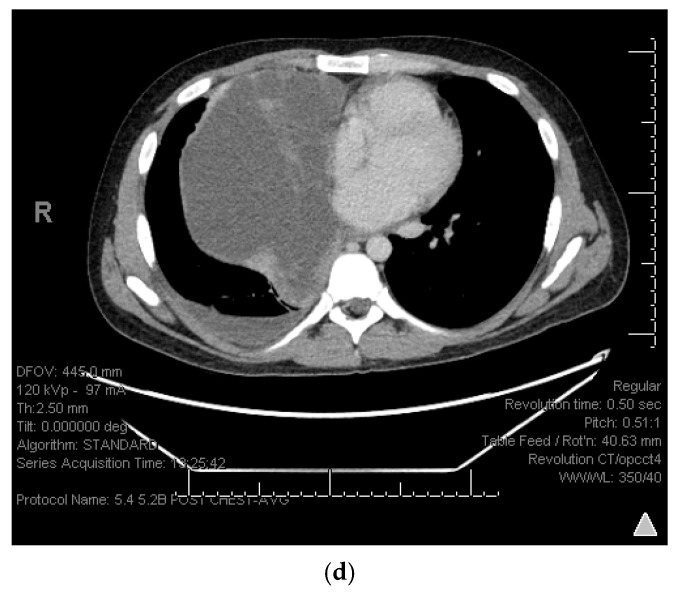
CT scans before and after chemotherapy in patients with primary lung synovial sarcoma. (a) Baseline CT scan. (b) CT scan after neo-adjuvant chemotherapy showing decreased size and increased necrosis. (c) Baseline CT scan. (d) CT scan after neo-adjuvant chemotherapy showing increased necrosis of the tumor.
